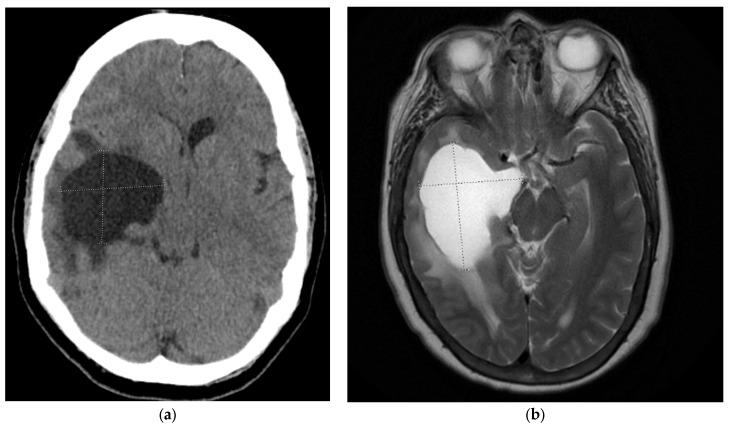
(a,b) Computed tomography (CT) head and T2-weighted magnetic resonance imaging (MRI) demonstrates a circumscribed cystic structure/lesion effacing the right temporal lobe with surrounding vasogenic edema and midline shift consistent with entrapment syndrome.
